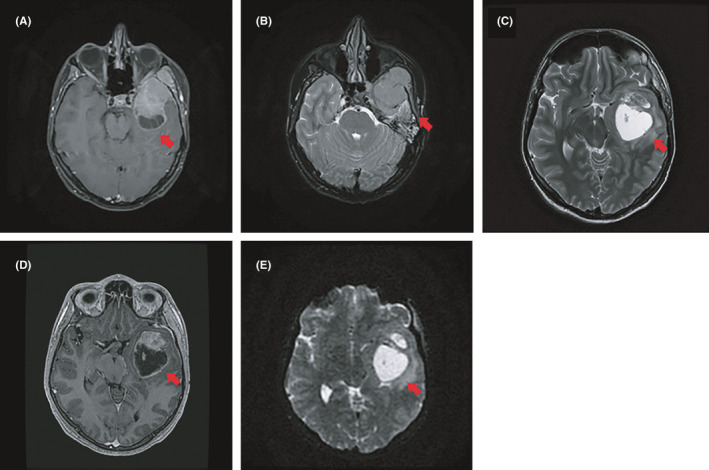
Axial MRI images A (T1W), B (T2W), C (T2 PROPELLER), D (FSPGR), and E (Diffusion weighted) demonstrating 7 cm by 4 cm lesion which consists of solid and cystic components with surrounding edema. Mass effect of the lesion causes midline shift of 6 mm to the right
